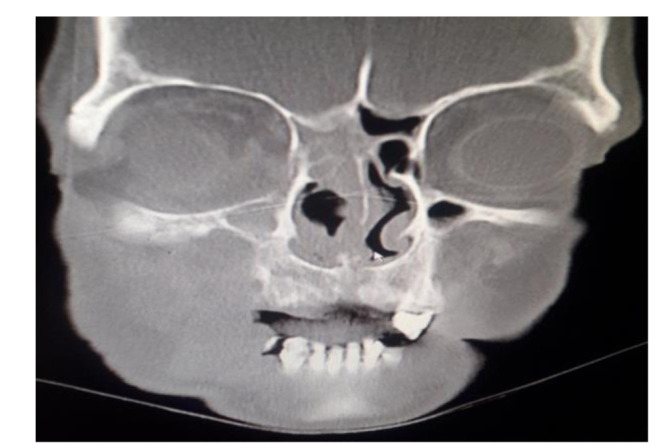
Paranasal sinus radiograph showing involvement of the right nasal cavity with the destruction of sinus walls
